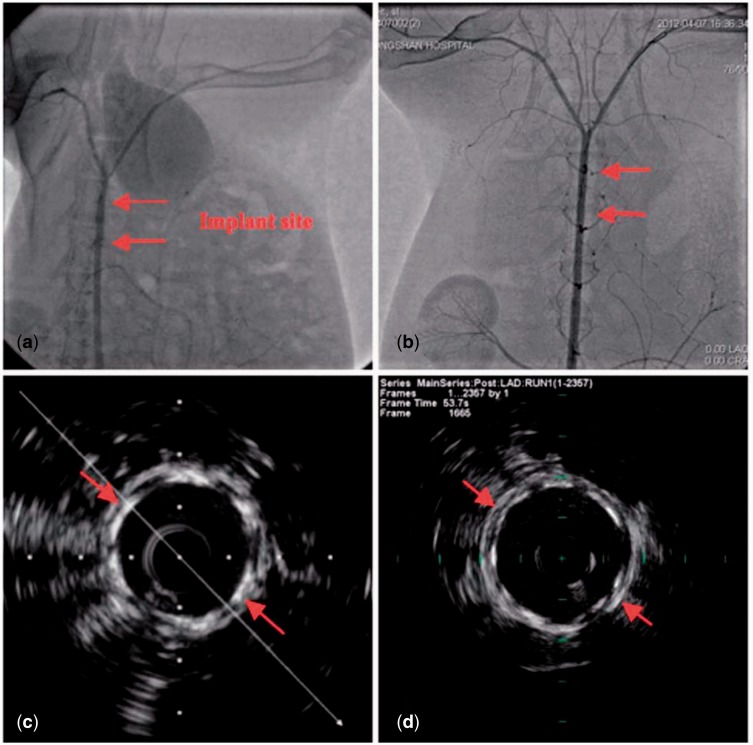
The as-implanted (a and c) and 16-week follow-up (b and d) angiographic and the corresponding longitudinal reconstruction Intravascular Ultrasound (IVUS) images of the abdominal aorta after JDBM stent implantation. Note the increased plaque volume and vessel size (arrow heads) at 16 weeks with the nearly complete absence of neointimal hyperplasia [26]
